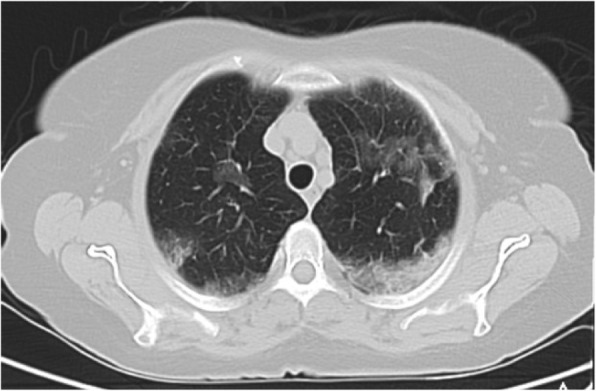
Simple pulmonary eosinophilia: thin section CT shows consolidation and GGO involving mainly the peripheral regions of both upper lobes
